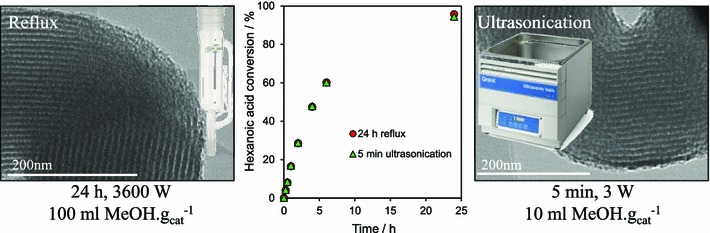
Surfactant template extraction via energy/atom-efficient ultrasonication delivers a one-pot PrSO_3_H-SBA-15 solid acid catalyst with identical structure and reactivity to that obtained by conventional, inefficient reflux. Adapted from reference [103] with permission from The Royal Society of Chemistry
